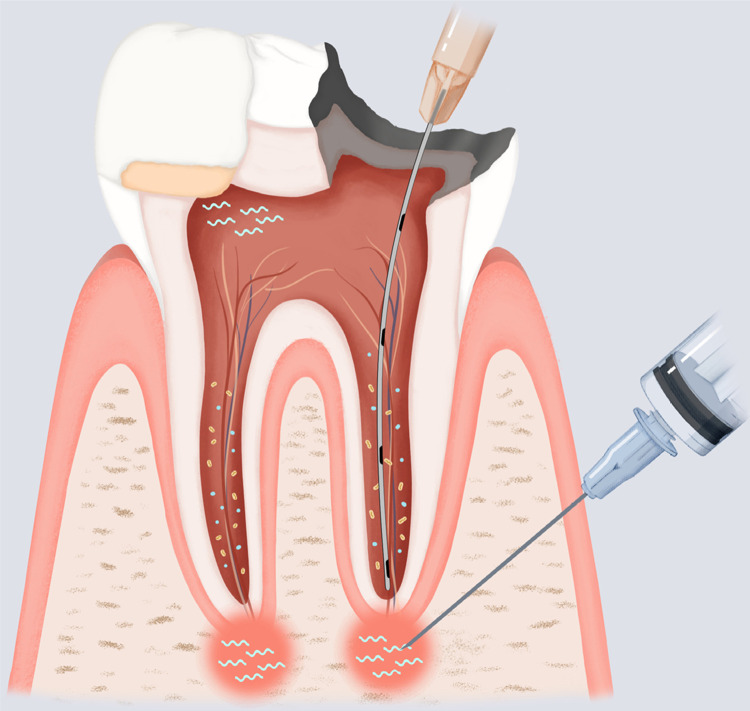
Potential clinical translation for the use of miRNAs in the treatment of pulpal and periapical diseases.
